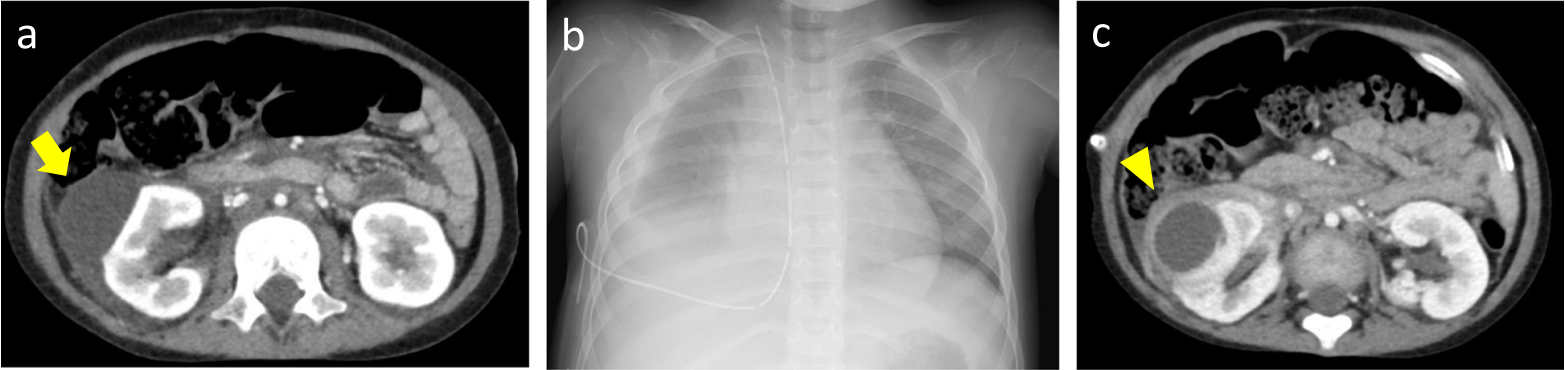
a Enhanced computed tomography showing a 4 cm size, nonenhanced, single, round and thin-walled cyst in the right upper kidney ten months before admission, indicating a simple renal cyst. b Chest X-ray showing massive pleural effusion on the right side on admission. c Enhanced computed tomography showing compression of the renal parenchyma, pelvis and ureter due to the enlargement of the right renal cyst and enhancement and thickening of the wall around the cyst, with perirenal inflammatory changes, on hospital day 21
